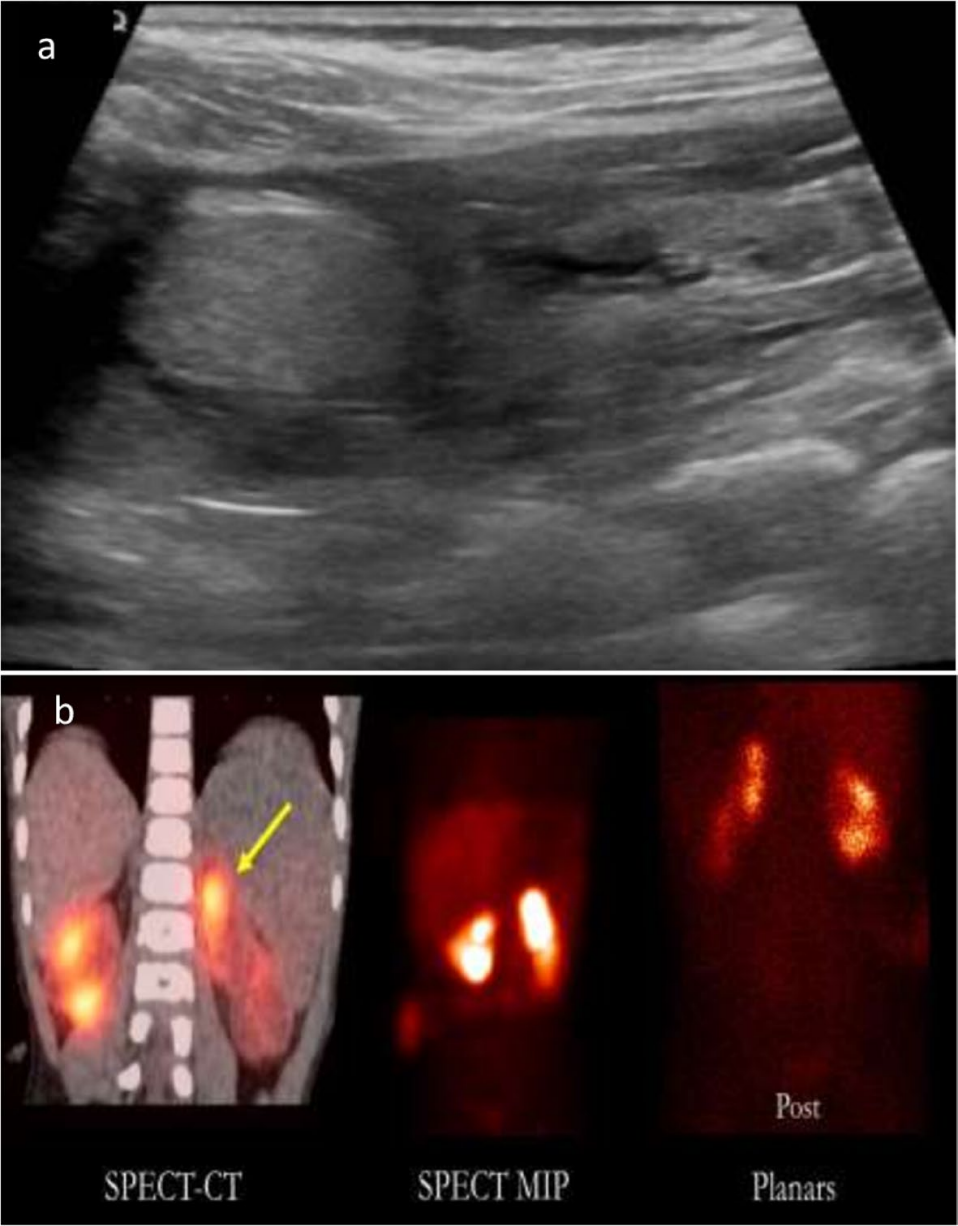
A US image of the left kidney shows an echogenic renal mass in the left upper pole. B 2-year-old boy with dysplastic kidneys and an echogenic left renal pseudo-tumor on US. The mass is best seen on 3D SPECT-CT and SPECT-MIP images compared to planar images showing radiotracer uptake and found to be a FPT. Split renal function was also decreased on the left to 37%
